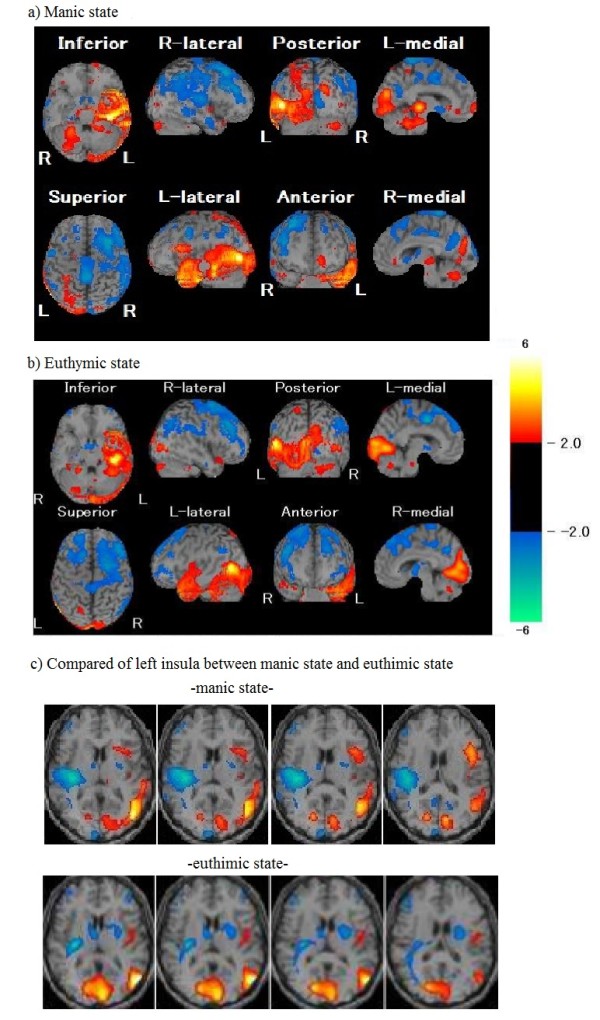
SPECT images obtained during a) the manic state and b) the euthymic state, and c) Comparison of regional cerebral blood flow of the left anterior insula between the manic state and the euthymic state.
